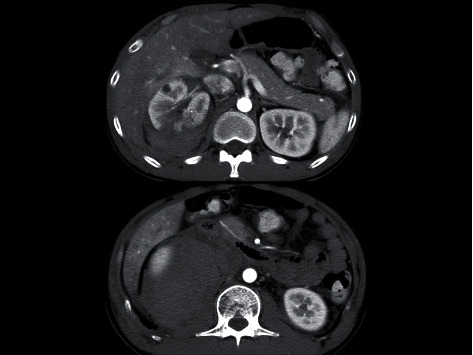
Enhanced abdominal computed tomography (CT) on hospital day 5. CT showed no exudative inflammation around the pancreas, which had been moved upward by the retroperitoneal hematoma.
